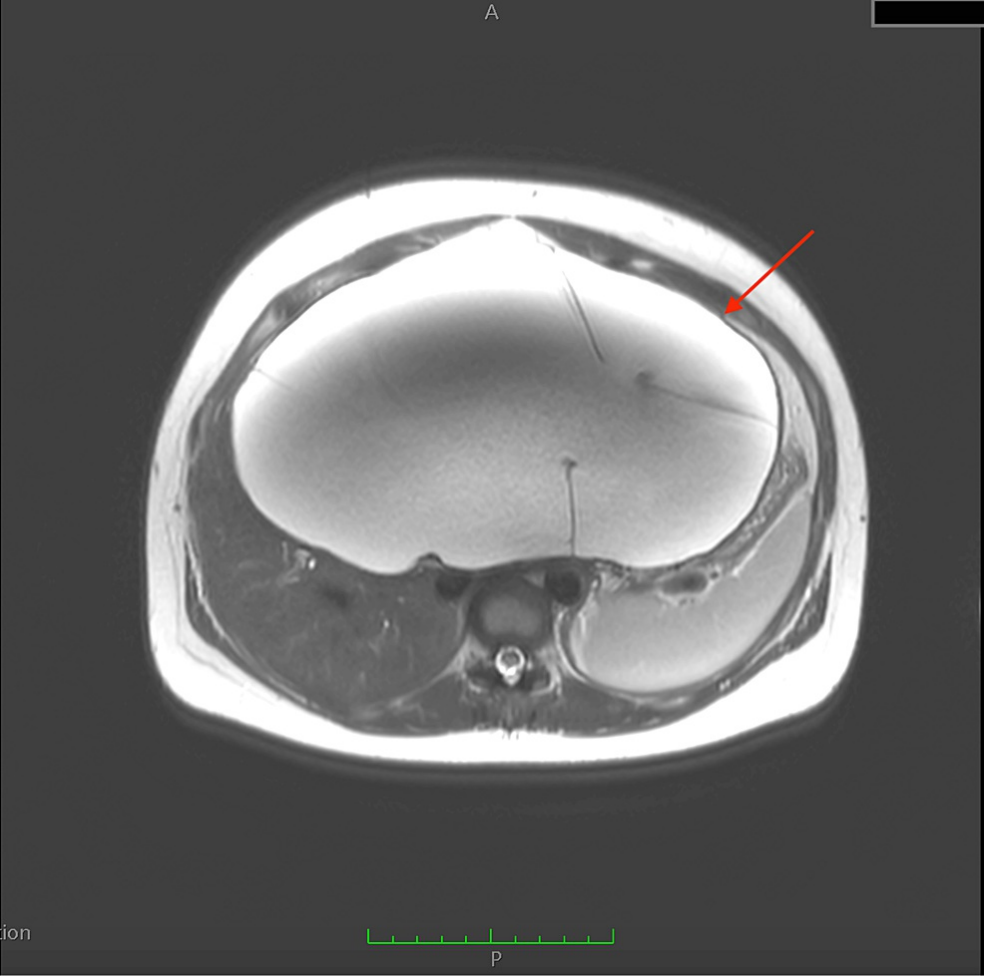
Preoperative MRI. Giant cyst, with a thin wall, in the left part of the liver with multiple septa. The presence of the giant cyst is indicated by the red arrow.
